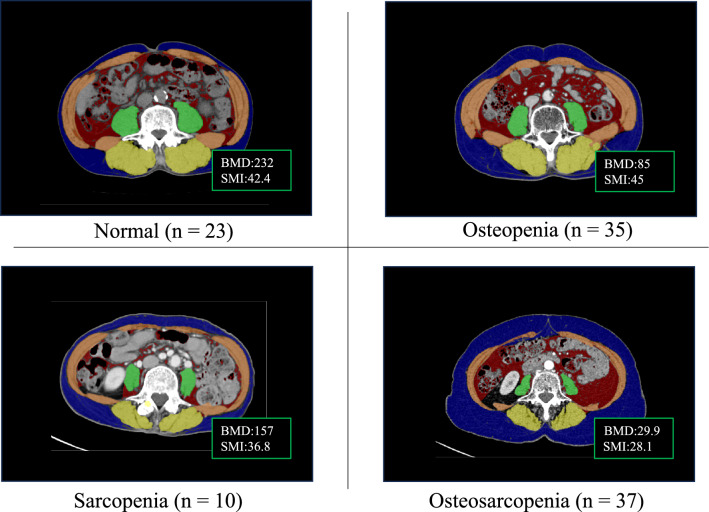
Representative computed tomography images of patients with normal, sarcopenia alone, osteopenia alone, and osteosarcopenia
